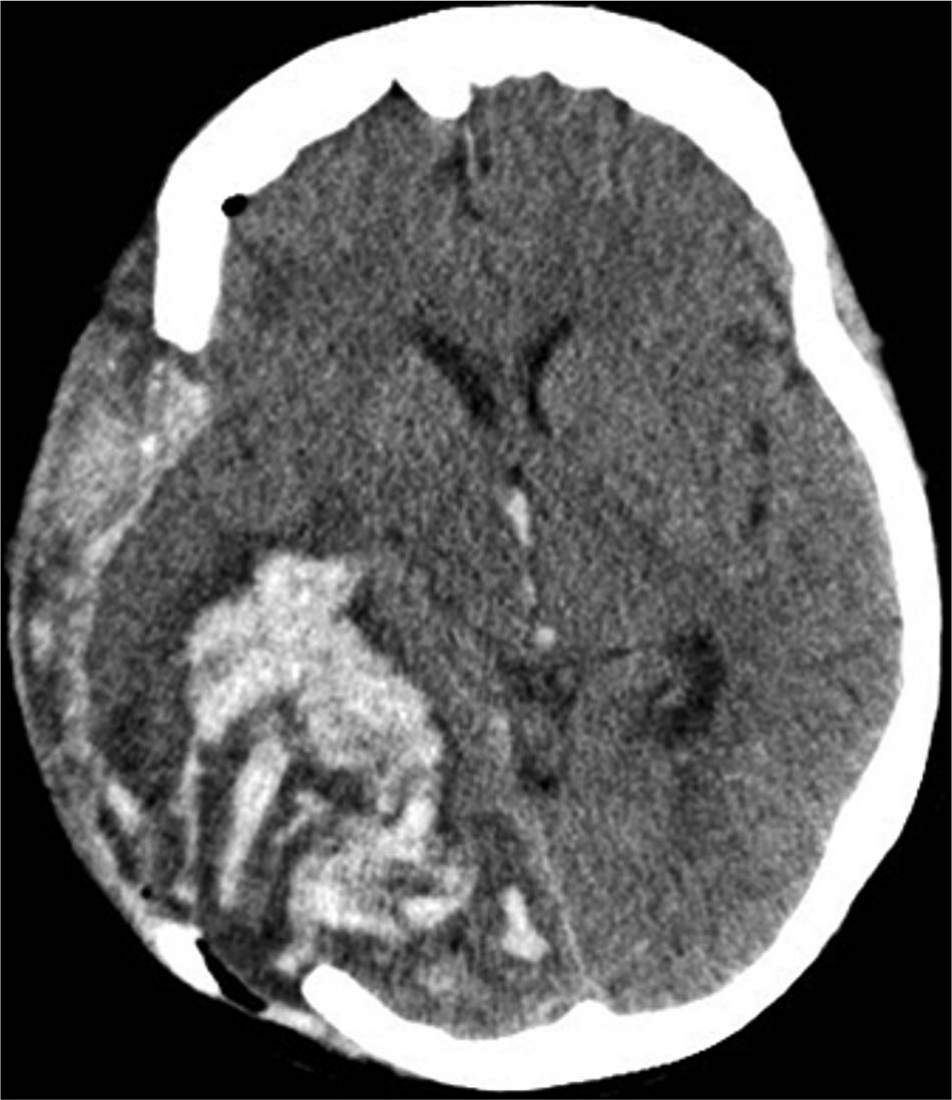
Postoperative axial CT scan. After osteoclastic craniotomy, a large bony defect with parenchymal hemorrhage and additional hemorrhage in the right parietal region is evident.
